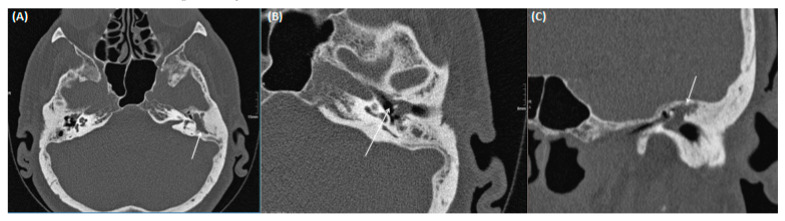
A 66-year-old male patient with pars flaccida cholesteatoma. Transversal (A,B) and coronal (C) CT using bone windowing. Complete soft tissue displacement of the epi-, meso-, and hypotympanum, but without bony destruction of the adjacent bony borders can be identified. Thickened tympanic membrane and beginning dissolution of scutum and ossicular chain are also present.
